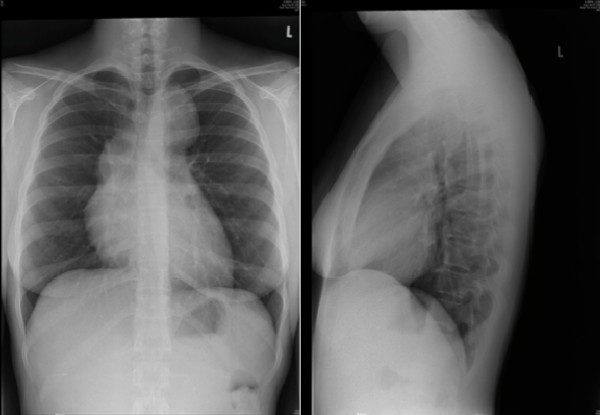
Chest x-ray, PA and Lateral views.
